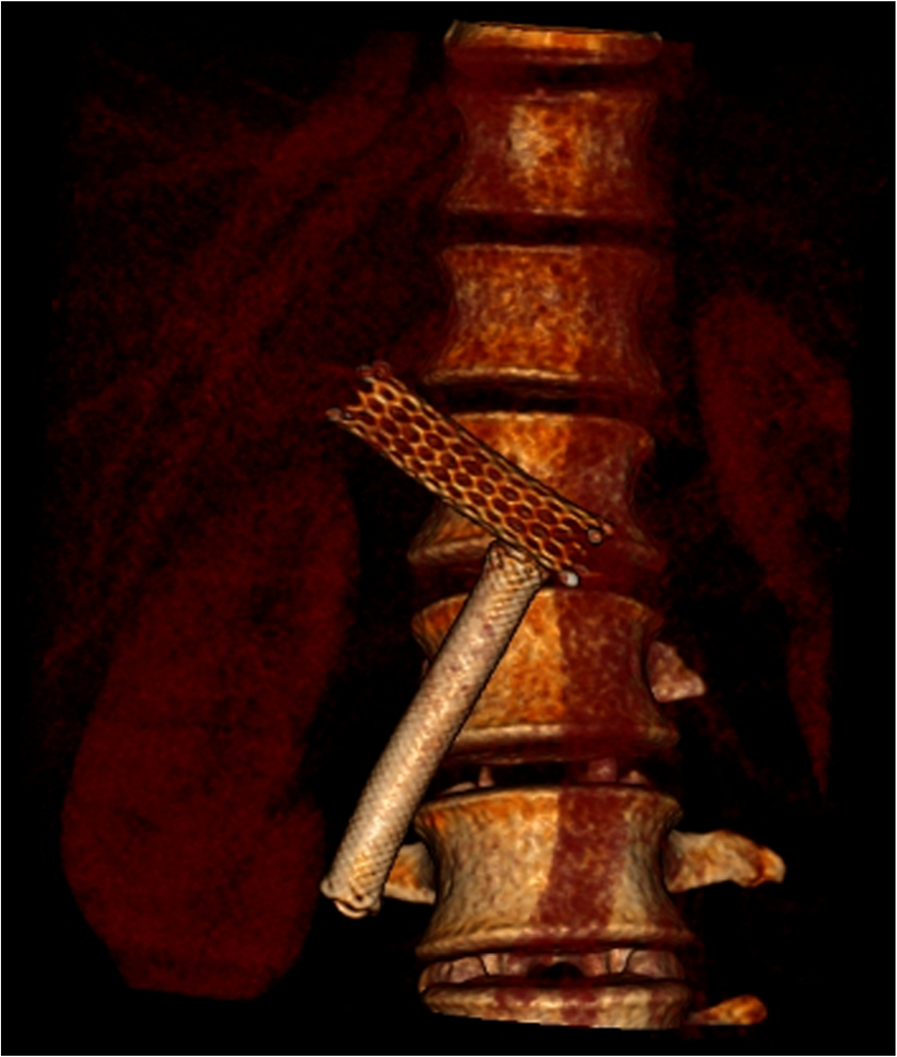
3d CT reconstruction of the covered vascular stent and the biliary stent. 3d reconstruction of a follow-up CT of the abdomen showing the relationship of the portal vein stent and the common bile duct stent. The covered portal vein stent has successfully excluded the pseudoaneurysm
